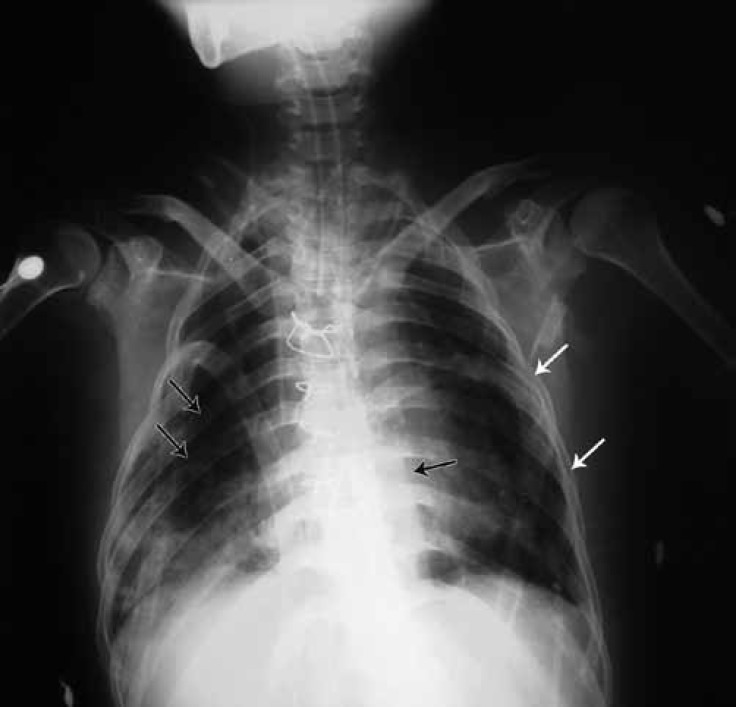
Chest x-ray of the patient (anteroposterior view) shows a small and bell-shaped thoracic cage (white arrows) with a round heart (black arrow in the middle). Thin ribs and slender long bones are also visible (black arrows on the ribs).
